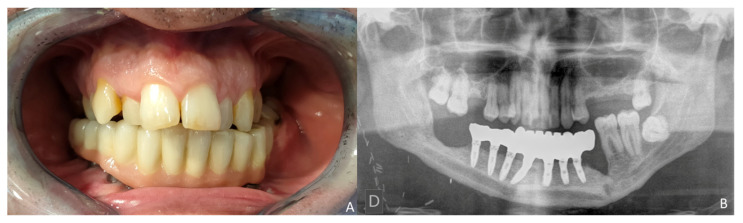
Functional result. (A) Final dental restoration. (B) Panoramic radiograph demonstrating the reconstruction of the previous height of the mandible with a correct osseointegration of the implants.
